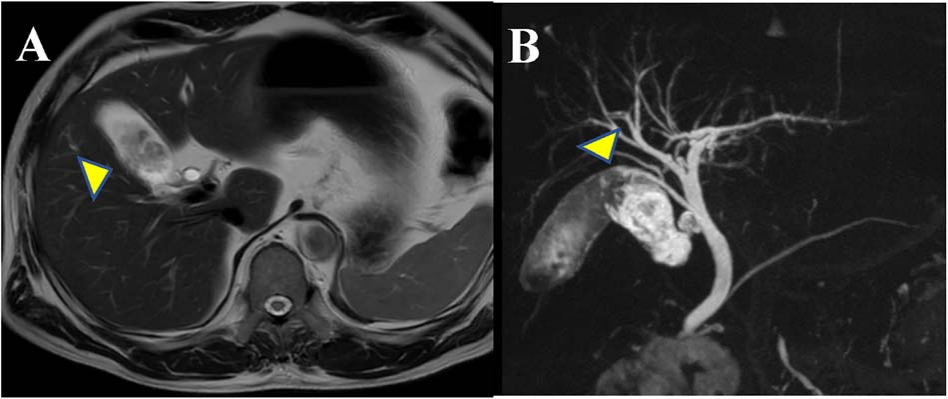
Findings of magnetic resonance imaging (MRI): (A) T2-weighted MRI (coronal image) shows the papillary tumor filling the body of the gallbladder. (B) T2-weighted MRCP image shows the accessory hepatic duct and it joins the common bile duct dorsally in the middle of the common bile duct.
